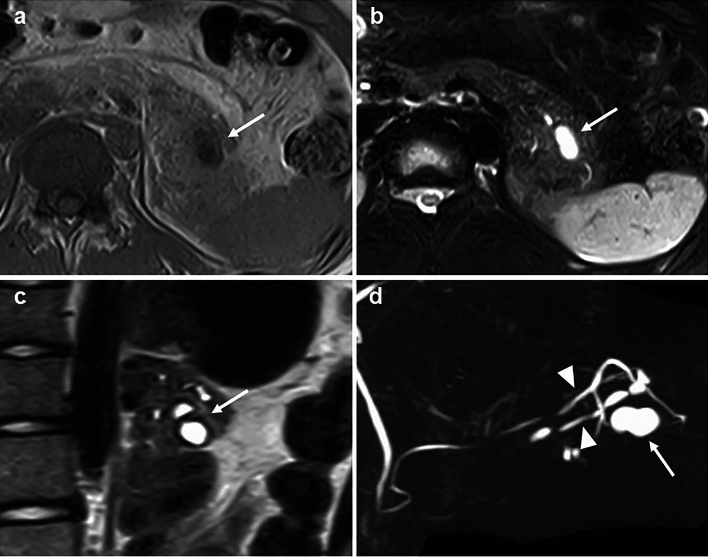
Magnetic resonance imaging. The cystic lesion (arrow) shows a hypointense signal on T1-weighted imaging (a) and a hyperintense signal on the T2-weighted image (b). The capsule shows a hypointense signal. The cyst was bilocular. (c). Magnetic resonance cholangiopancreatography (d) shows that the pancreatic tail cyst communicated with the main pancreatic duct and appeared to be two MPDs in the pancreatic tail (arrowheads)
